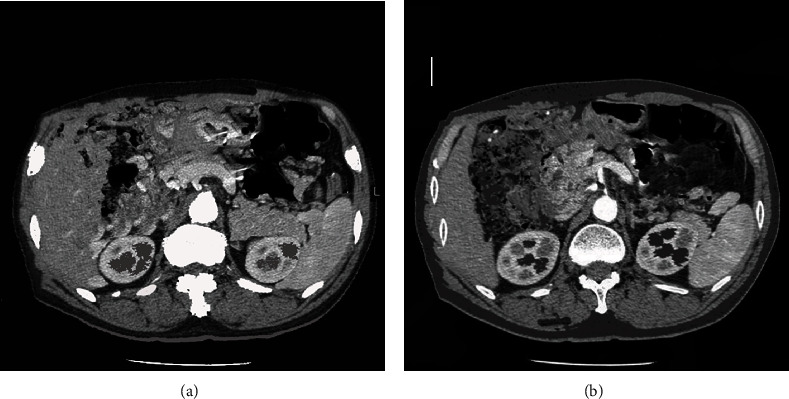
CT images of leiomyoma and mesenchymal tumor. (a) CT image of leiomyomas (low-density lesion with complete mucosal line); (b) CT image of mesenchymomas (internal bleeding with uneven enhancement).
